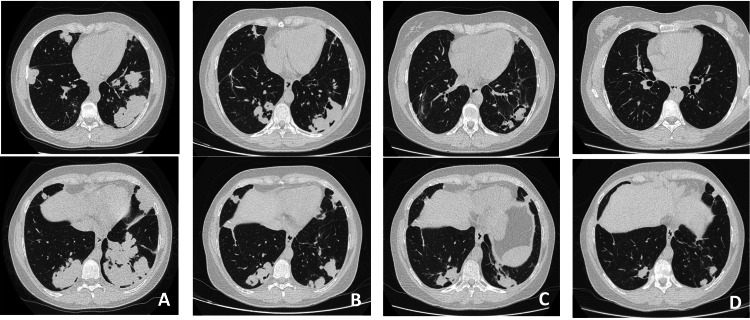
Chest computed tomography evolution Evolution of the imaging pattern on chest computed tomography - at admission (A), one month after starting immunosuppression with methotrexate and steroids (B), two months and 18 months after taking rituximab (C, D, respectively).
